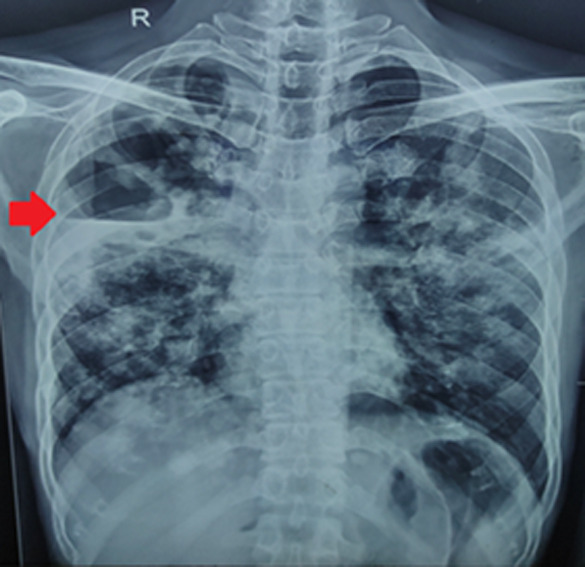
chest X-ray posteroanterior view showing bilateral lung consolidation with a cavitary lesion in the right upper zone (red arrow), with an air-fluid level, suggestive of a lung abscess
